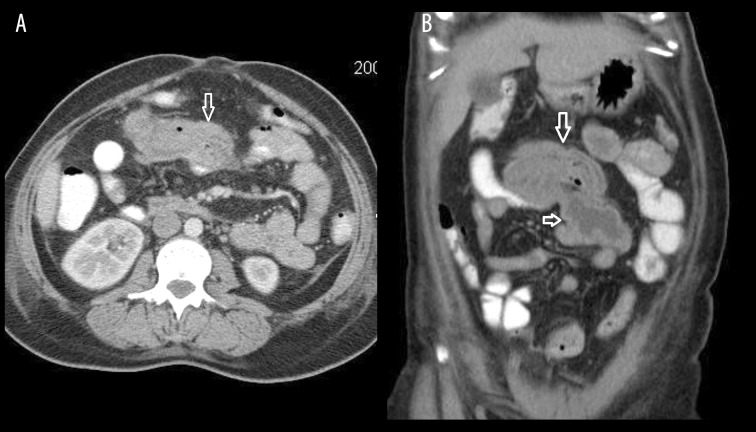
(A) Axial contrast-enhanced CT scan at the level of the upper pole of right kidney reveals inhomogeneous mass with gas bubbles within, caused by a retained sponge. (B) Coronal reformatted image shows the retained sponge, causing mass effect on an adjacent small bowel loop.
